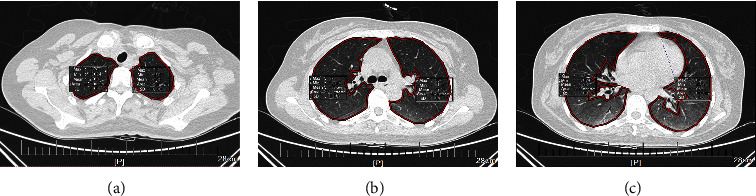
The 3 representative levels of chest HRCT. (a) Carinal plane, (b) 5 cm plane above carina, (c) 5 cm plane below carina.
